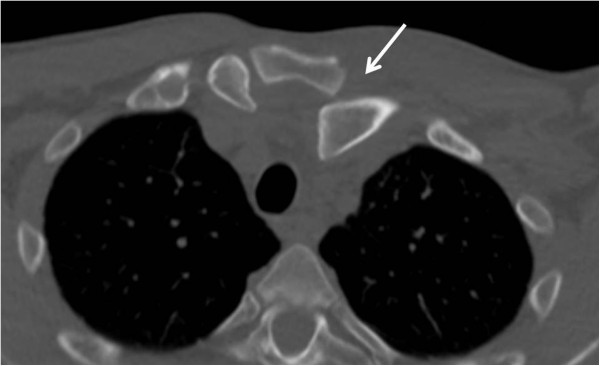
The axial CT scan confirms the clinical suspicion of a left posterior sternoclavicular joint dislocation (arrow).
